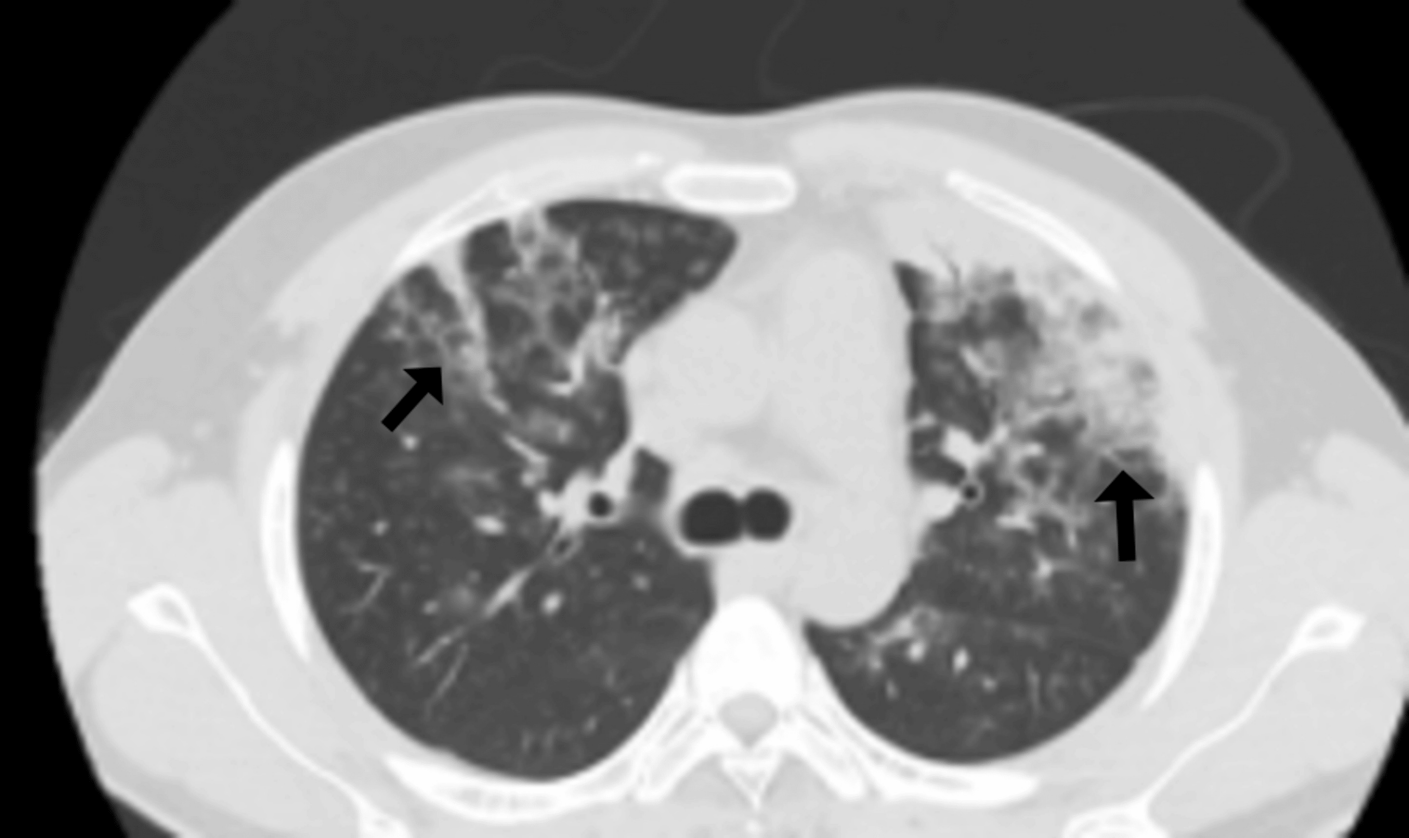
High-resolution computed tomography (HRCT) scan of the chest. Bilateral segmental and subsegmental consolidations with air bronchograms, along with peripheral patchy ground-glass opacities and atelectatic bands, were observed in the upper and middle lobes (black arrows).
